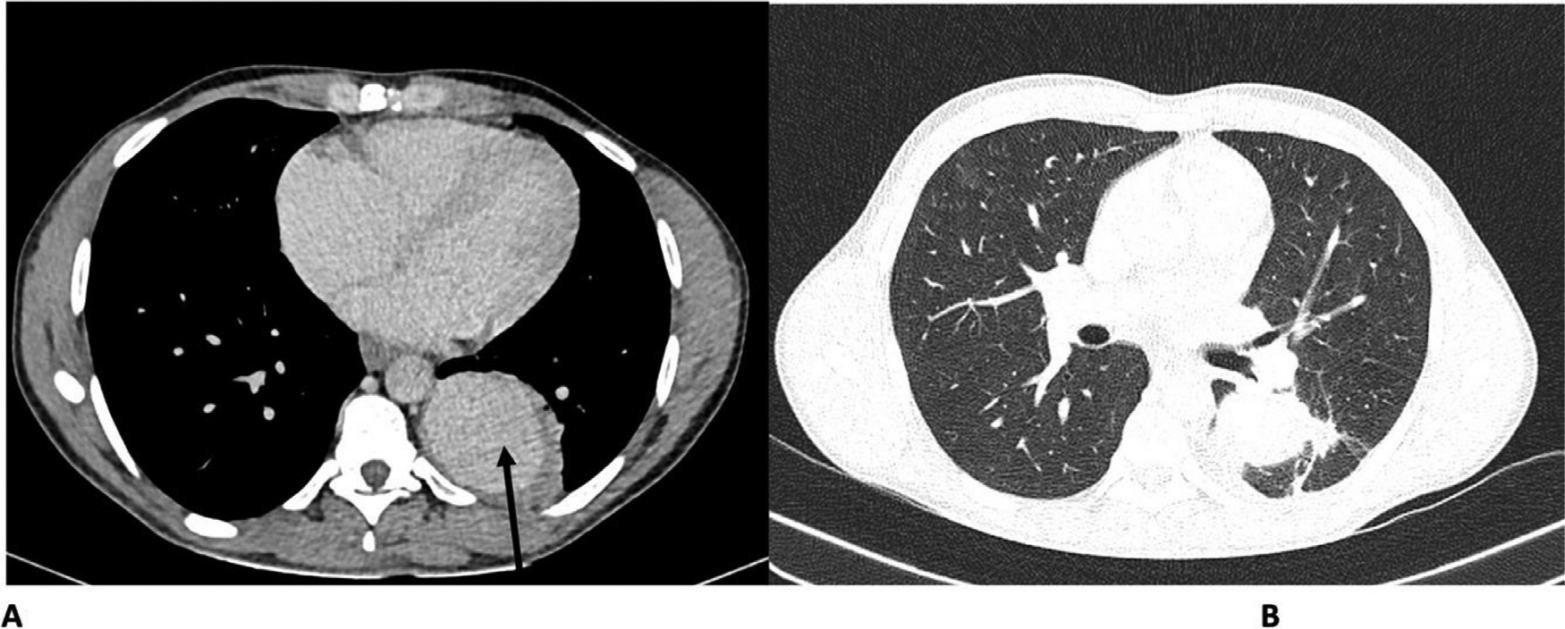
Two months post-treatment CT scan. Contrast-enhanced chest CT scan shows a reduction in the size of the aneurysm (solid arrow in A), measuring 5.8 (TRV) x 5.3 (AP) x 6.8 (CC) cm, with complete resolution of the adjacent left lower lobe cavity. Partial resolution of the left lower lobe consolidation is also seen with reticulations in the superior segment of the left lower lobe suggesting fibrotic changes as seen in B.
